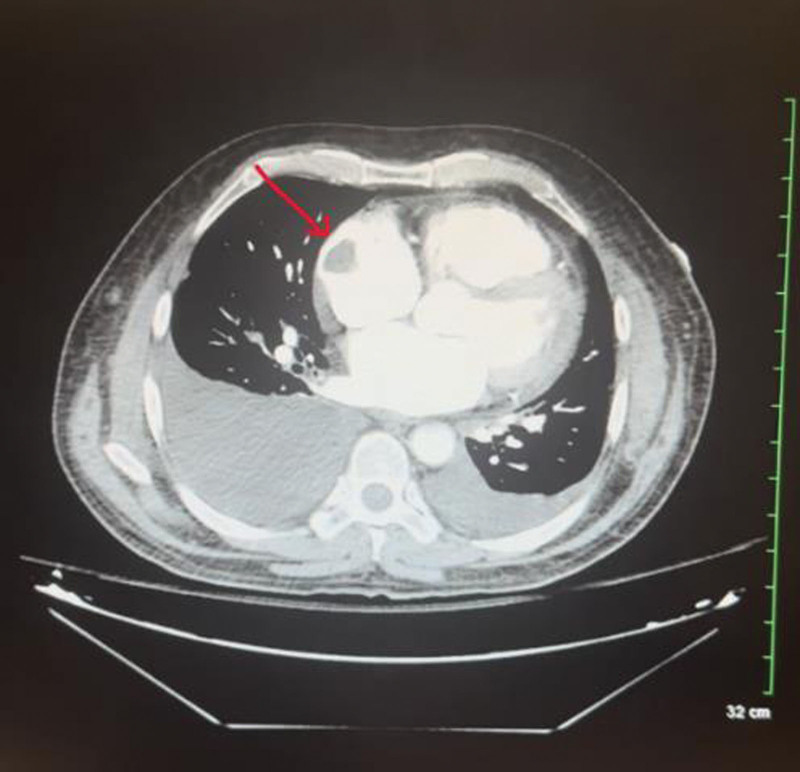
A CT scan image showing a large filling defect visible in the RA. CT = computed tomography, RA = right atrium.
